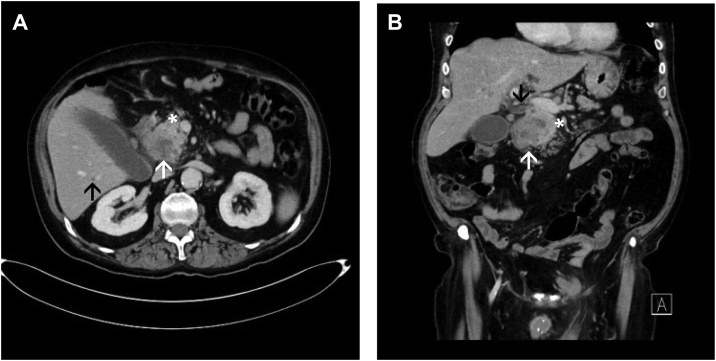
Preoperative computed tomography (CT). Inhomogeneous hypodense ill-defined focal lesion of the head of the pancreas (white arrows) with a dilated intra- and extrahepatic bile duct system due to a mass effect (black arrows) (A transverse image, porto-venous phase; B coronal image, porto-venous phase). Note the healthy adjacent parenchyma of the pancreas (white asterisks) and lack of signs of local irresectability.
